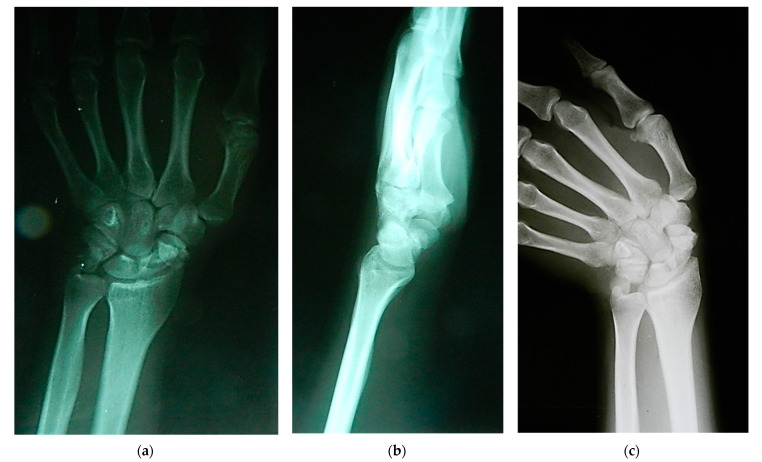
Radiographs showing scaphoid nonunion: (a) posteroanterior; (b) lateral; and (c) scaphoid radiographic views.
